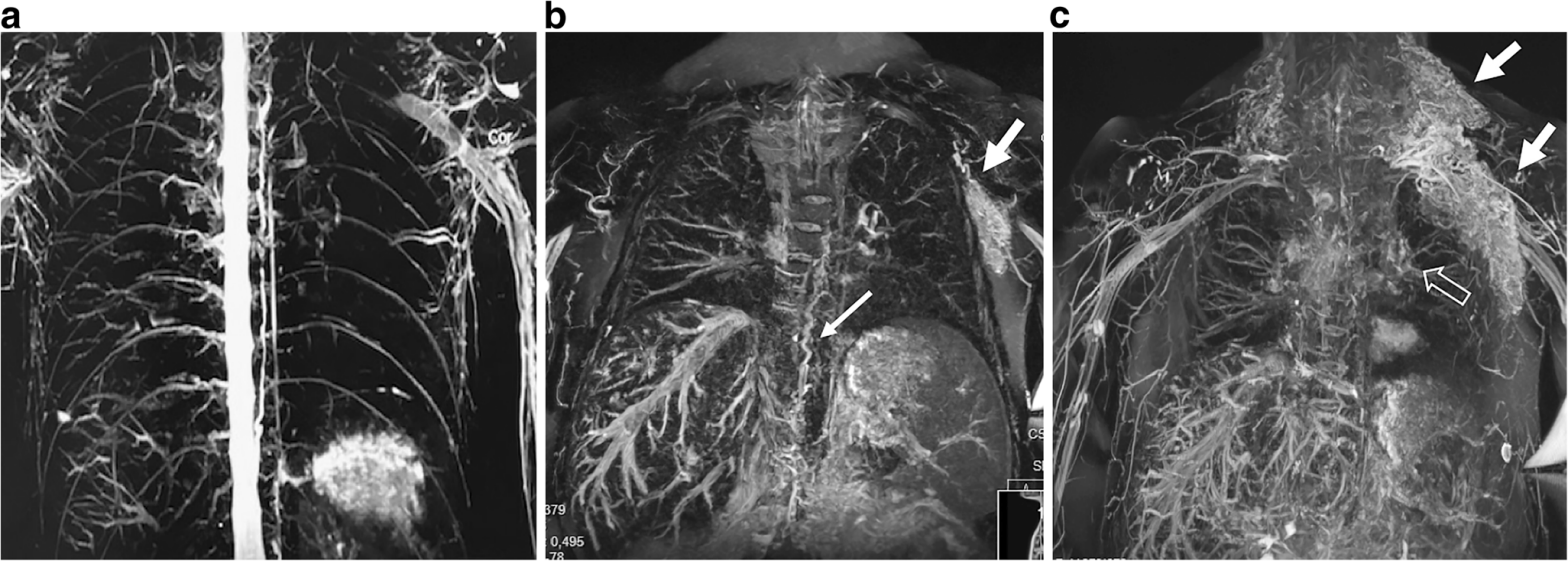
Magnetic resonance lymphography. a–c T2-weighted coronal MR sequence in a healthy control subject, a 25-year-old woman (a), and in a Fontan-operated 16-year-old boy (b, c) with findings of a tortuous thoracic duct (*thick arrows* in b). Note the lymphangiectasis in the supraclavicular and axillar regions on the left side (*solid arrows* in b and c) and perihilar region (*open arrows* in c). There is additional perivenous edema in the liver and mesentery (b)
